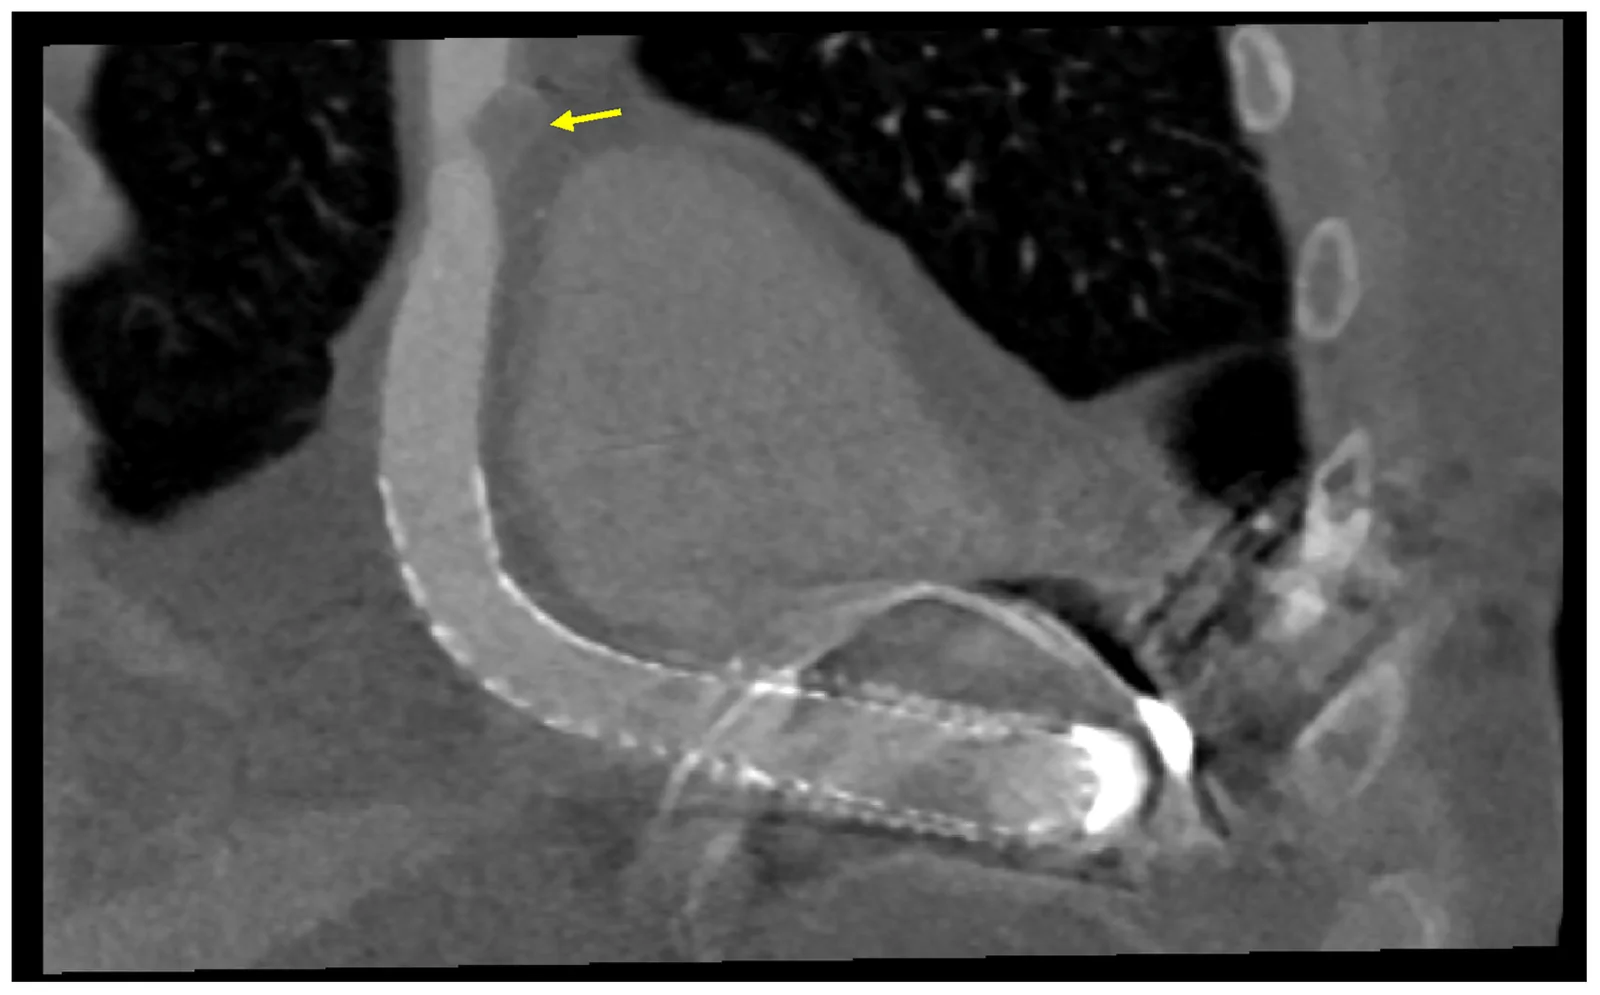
Follow-up computed tomography angiography (CTA) was obtained, showing patent proximal stents and a remaining short-segment thrombus (*yellow arrow*) distal to the stents at the anastomosis to the ascending aorta.
